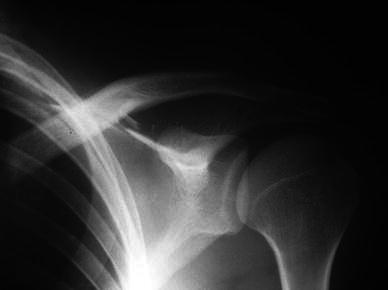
X-ray of the shoulder showing an avulsion of the scapular spine
